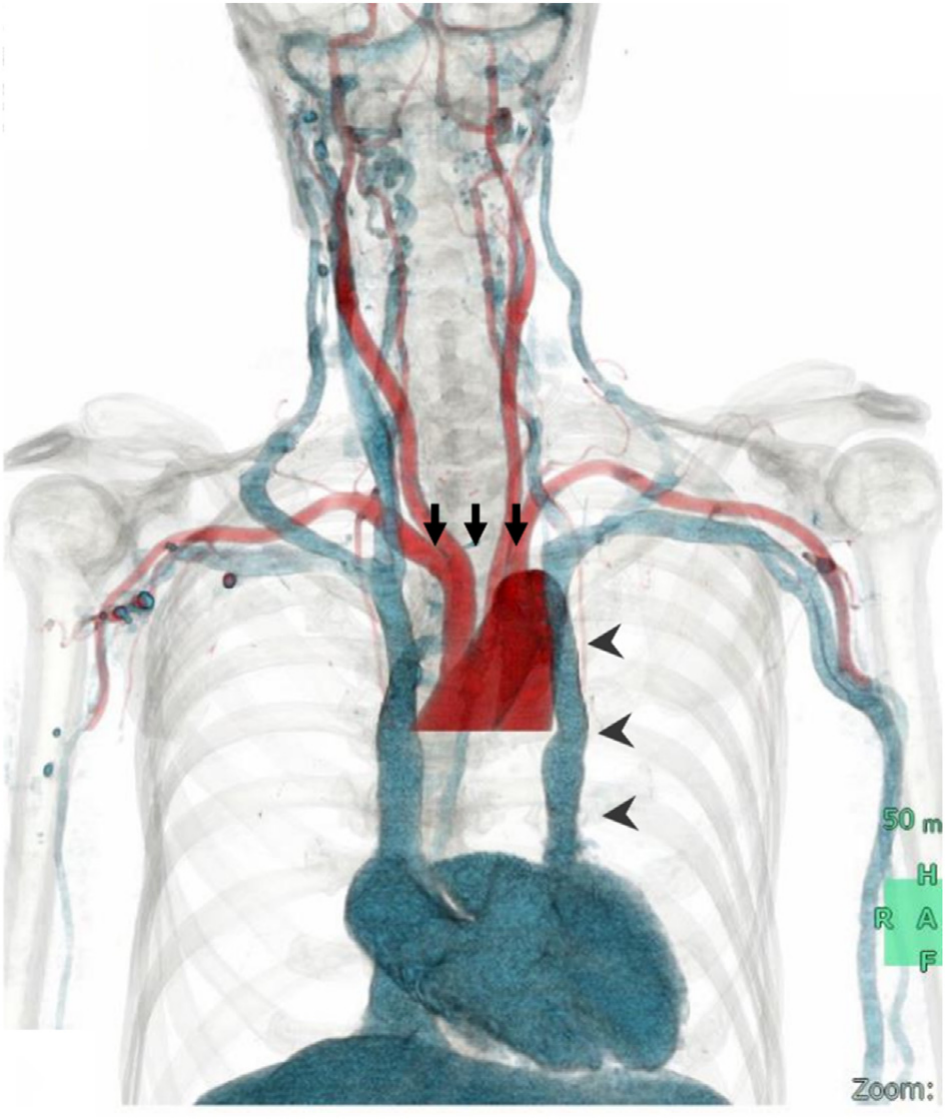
Contrast-enhanced computed tomography demonstrating absence of connection between the right and left brachiocephalic veins (arrow) and presence of left superior vena cava coursing down the left mediastinum and draining into the heart (arrow head).
